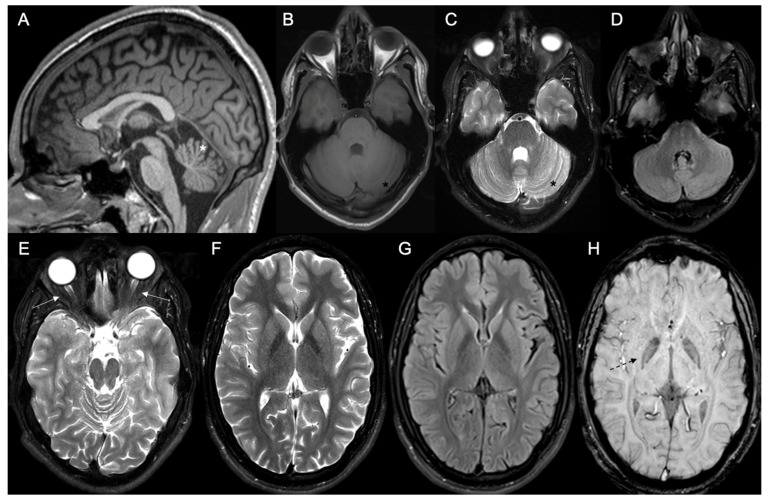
Brain MRI study of the proband at age 18 years old. (A) Sagittal brain MRI showing cerebellar atrophy (white asterisk) and normal corpus callosum in T1-weighted sequence. Axial brain MRI disclosing atrophy of the vermis and cerebellar hemispheres (black asterisks) in T1 (B), T2 (C), and FLAIR sequences (D). (E) Axial brain MRI showing mild atrophy of the optic nerves (white arrows) in T2-weighted imaging. No basal ganglia signal changes were observed in T2 (F) and FLAIR sequences (G). (H) Axial brain MRI showing hypointense sign in medial globus pallidus (black arrow) in SWI sequence.
